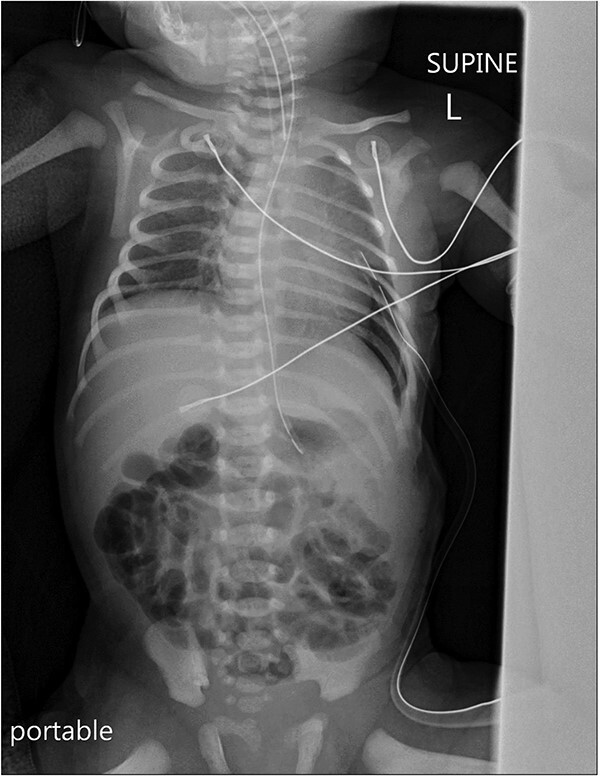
A portable X-ray photo of the chest, abdomen and pelvis taken postoperatively shows the expansion of both lungs and midline mediastinal position. A small subpulmonic pneumothorax on the left side is visible, for which a chest tube was inserted.
